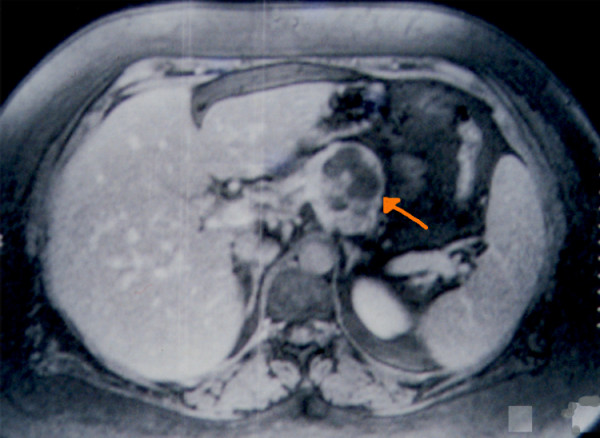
MRI showing cystic and solid lesion in the pancreas.
